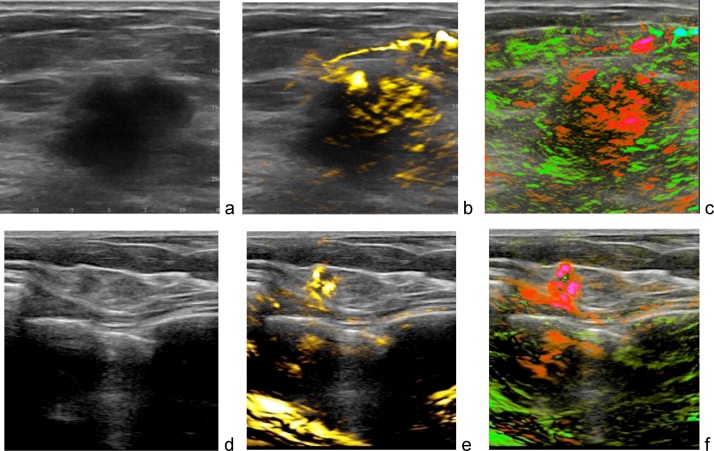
Two examples of OA/US images of breast carcinoma in two different patients. Upper row shows a 2.6 cm malignant mass on gray scale ultrasound (a) with increased internal total hemoglobin due to high density of angiogenesis in more than half of the tumor (b) and diffuse internal blood deoxygenation (c). The bottom row shows a small 4 mm grade II invasive ductal carcinoma with relatively low contrast on gray scale ultrasound (d), however with high density of angiogenesis around a small core on OA total hemoglobin map (e) and significant blood hypoxia on [sO2] map. Note that there is dense angiogenesis microvasculature with significant deoxygenation (red) within these masses and their margins. Also, the upper row Fig.12c shows numerous green radiating parasitized feeding arteries in the tissues that surround the mass – additional image feature indicating pathology of malignancy. Images are 38 × 38 mm.
